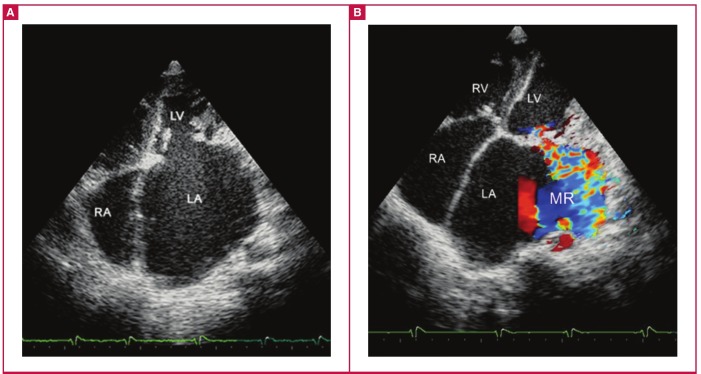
Basic echocardiographic features of left ventricular EMF. A and B, apical four-chamber view. The huge left atrium with apical and LV wall fibrosis, obliterated LV and mitral regurgitation (MR) (B), are the characteristic features of left ventricular EMF.
